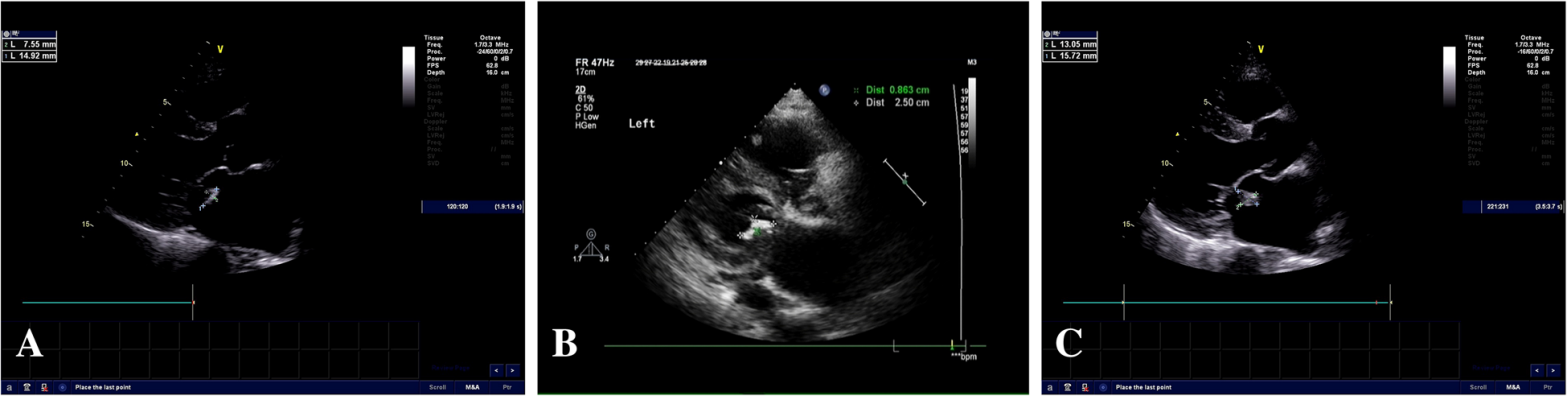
Transesophageal echocardiography showing the vegetation (red arrow) on the posterior leaflet of the mitral valve at different times. a October 11, 2018; b November 6, 2018; c October 22, 2019
